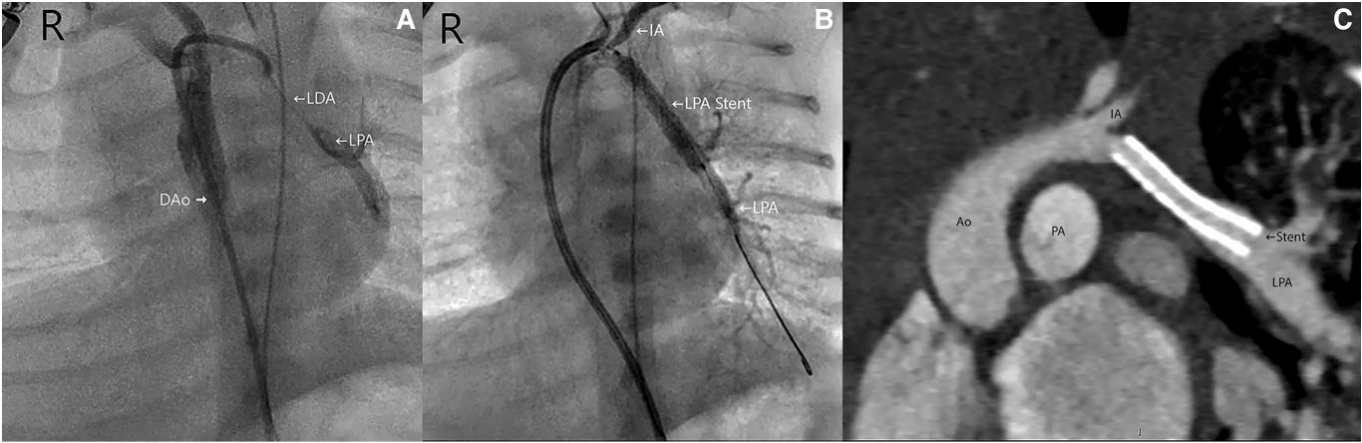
(*A*) Anteroposterior view of angiography showing thread-like LDA connecting from left innominate artery to LPA. (*B*) LPA stent re-establishes flow to distal LPA (see Supplementary material online, *Video S2A* and *B*). (*C*) CT angiography repeated 2 months post-stenting. The stent was patent, with good growth of distal LPA. DAo, descending aorta; LDA, left ductus arteriosus; LPA, left pulmonary artery; IA, innominate artery; Ao, aorta; PA, pulmonary artery.
